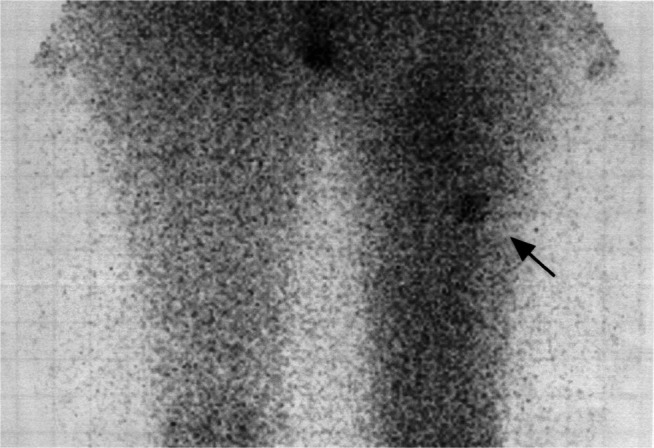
The patient had a local relapse of right-thigh aggressive fibromatosis: a scintigraphy with ^111^In-labelled octreotide (OctreoScan) revealed tumour uptake of the radiopeptide (arrow).
